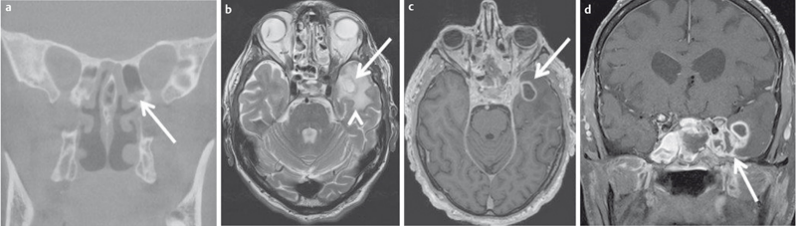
67-year-old diabetic patient with numb centrally located headache. a) Coronal reconstructed DVT demonstrates inflammatory changes of the posterior ethmoid cells (arrow) and the sphenoid sinus on the left side. b) New onset of seizure a few hours after the DVT. T2w MR images demonstrate a ring-like mass (arrow) with surrounding edema (arrowhead). c) The lesion displays homogenous ring-like enhancement on axial T1w images. d) Contrast-enhanced coronal T1w images demonstrate extension to the dura and the thrombosed cavernous sinus. Fungal sinusitis was confirmed by surgery.
